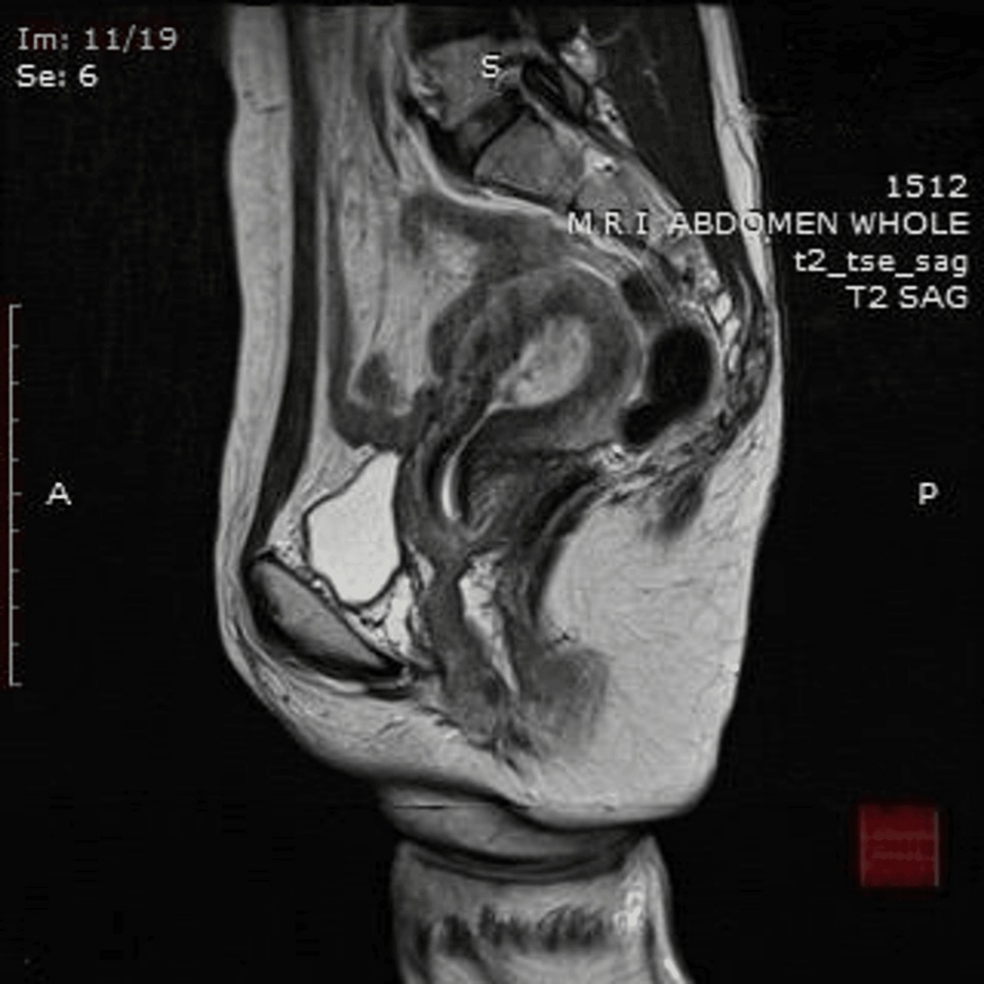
MRI suggestive of uterine arteriovenous malformation The image depicts features likely suggestive of enhanced myometrial vascularity with hypervascular (type III) retained products of conception. MRI, magnetic resonance imaging
